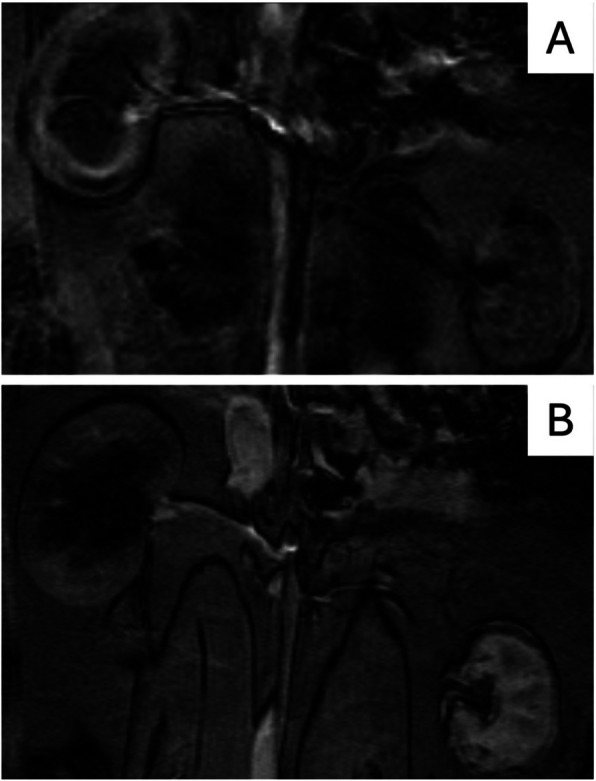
MRI done one month (M1) after embolization of the left renal artery with 25% Glubran®2. A coronal T1 view after contrast injection at the early arterial phase: persistent occlusion of the left renal artery with no parenchymal enhancement. B coronal T1 view at the late venous phase: parenchymal enhancement due to subcapsular neovascularisation
